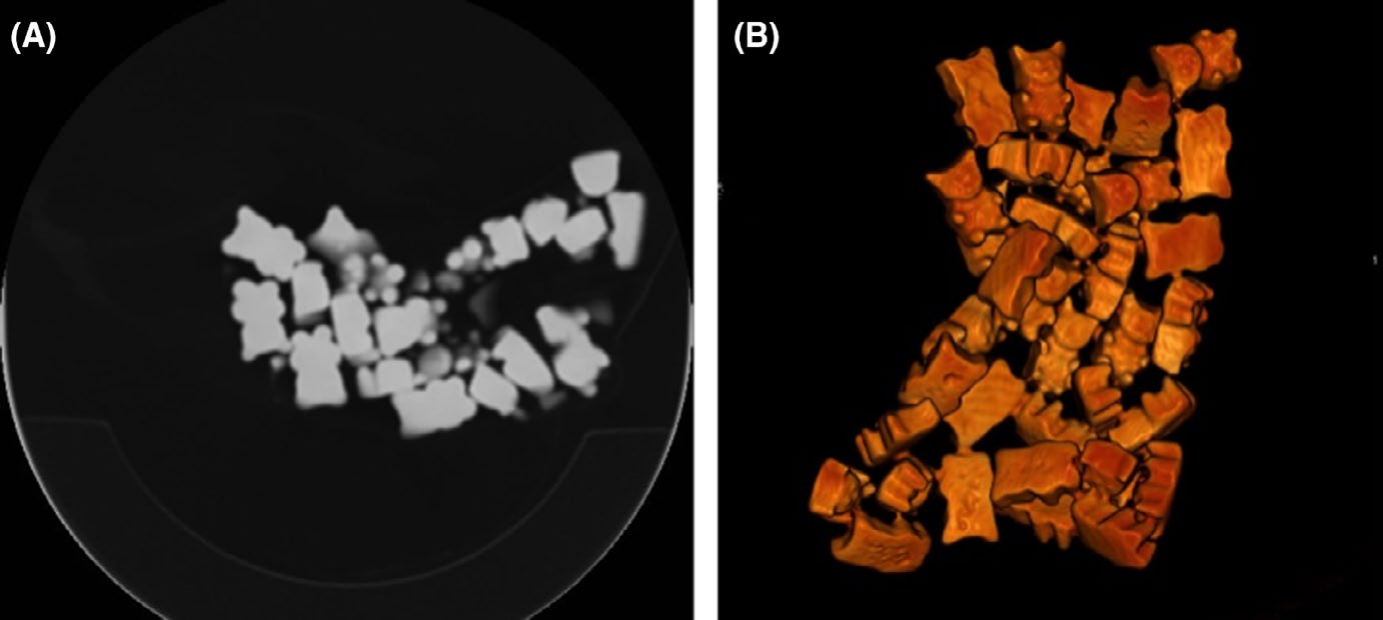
Noncontrast‐enhanced CT of a bag of gummy bear sweets, axial (A) and 3D reconstruction (B). Note the spontaneous dense aspect of the sweets
